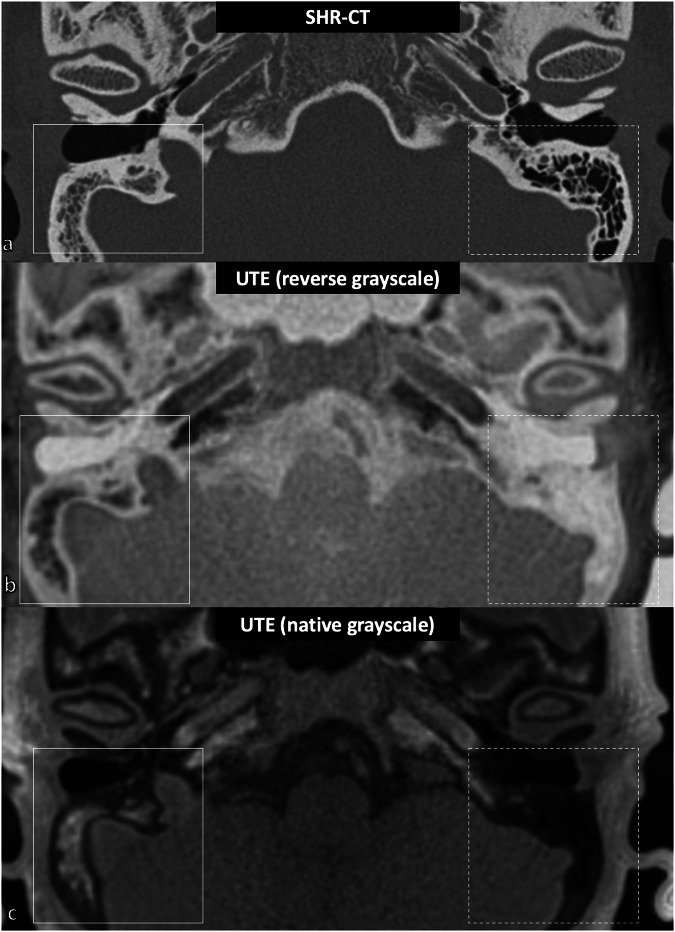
Pneumatization of mastoid air cells in SHR-CT and UTE-MRI (example in living patient) in the axial plane. a Mastoid air cells in SHR-CT. Note the hypopneumatization of the right mastoid air cells (white box), whose bony appearance is comparable to the zygomatic process of the temporal bone, and the standard pneumatization of the left mastoid air cells (dotted box), with their corresponding aspects in the UTE-MRI sequence with reverse grayscale (b) and native grayscale (c). CT, Computed tomography; MRI, Magnetic resonance imaging; NR, Normal resolution; SHR, Super-high resolution; UTE, Ultrashort time of echo
